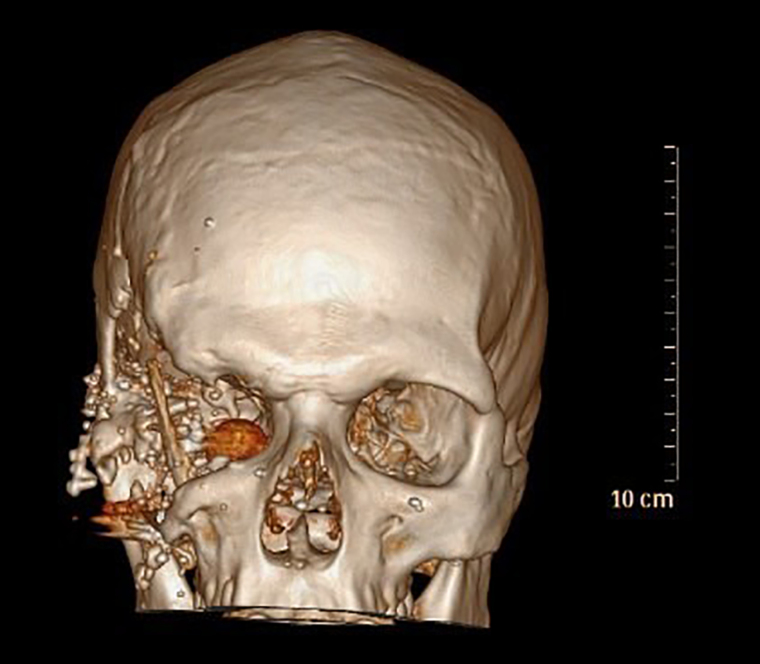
Postoperative 3D CT reconstruction (frontal view) demonstrating the restoration of the right lateral orbital rim with stable positioning of the iliac crest bone graft. 3D = three-dimensional, CT = computed tomography.
